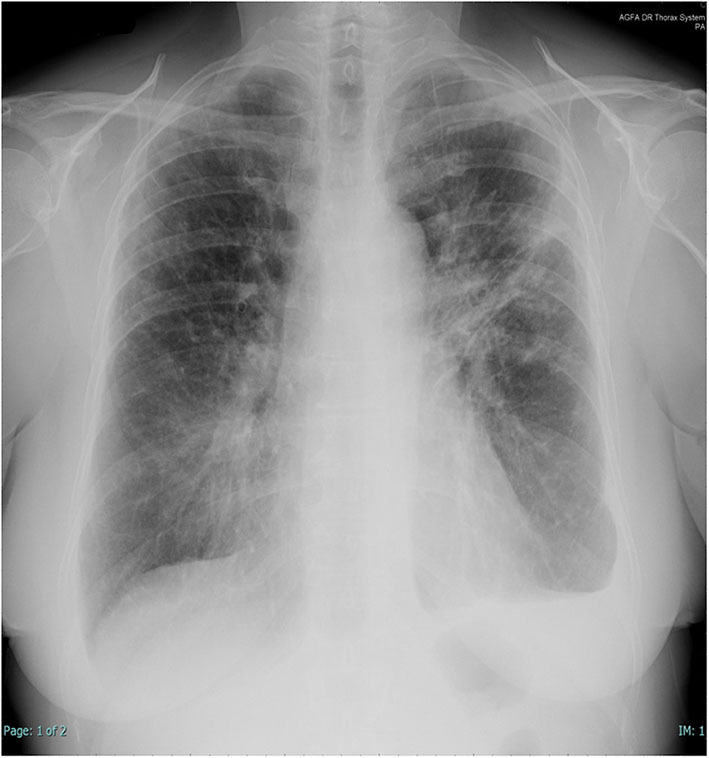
Chest X-rays at presentation. Left subclavian pulmonary thickening associated with patchy bilateral peripheral opacity particularly represented in the left lung where there are also band-like consolidations and pleural effusion.
